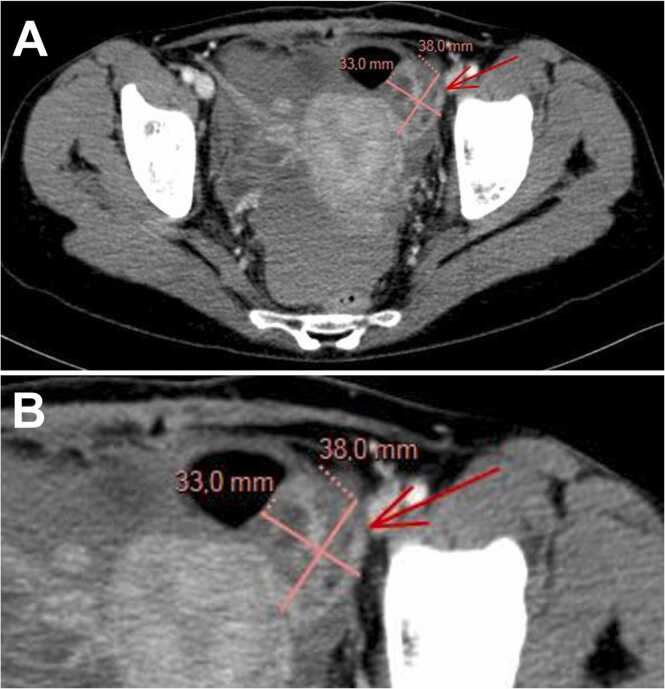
Abdominopelvic computed tomography (axial soft-tissue window). (A) Overview showing a multiloculated left latero-uterine adnexal mass (arrow). (B) Magnified view demonstrating thin septations with approximate diameter of 38 × 33 mm. No ascites and no visible pelvic lymphadenopathy.
